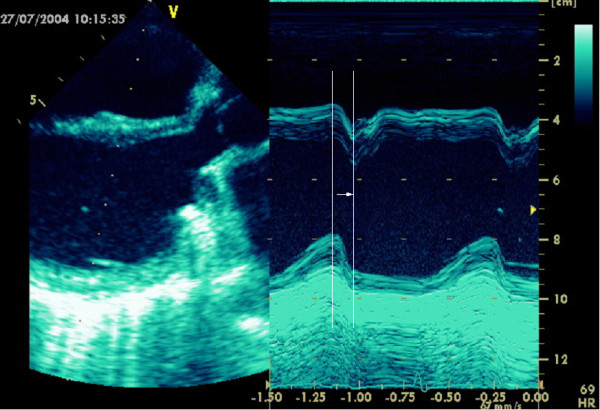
Assessment of asynchrony in parasternal long axis view by M-mode: Time difference between peak of septal and inferior myocardial contraction.
